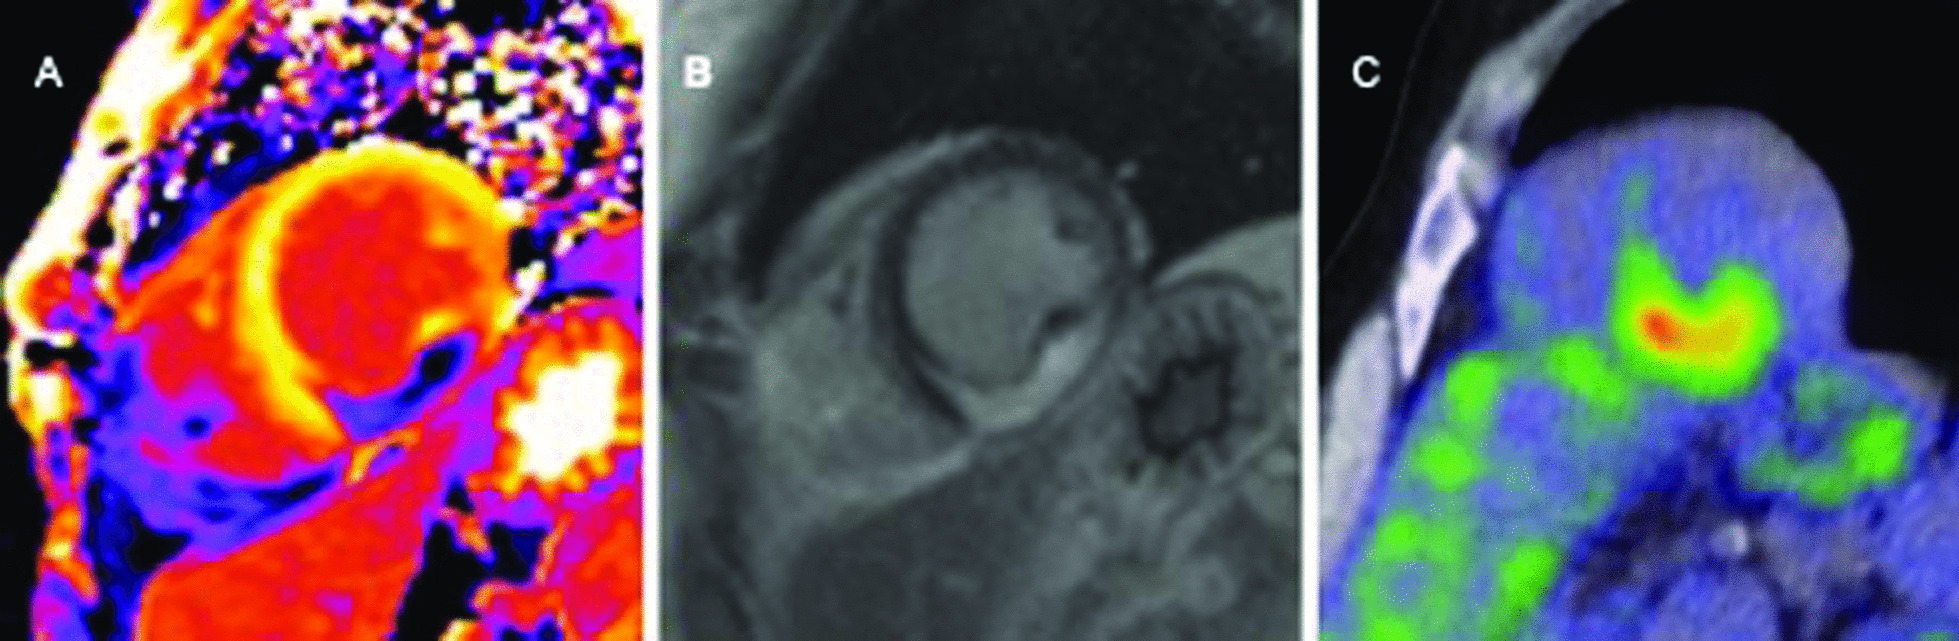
Example for determination of active cardiac sarcoidosis. Imaging findings in one patient with cardiac sarcoidosis (CS) in cardiovascular magnetic resonance (CMR) and 18-fluorodeoxyglucose positron-emission tomography-computed tomography (PET-CT). A Shows post contrast T1-map, B typical intense intramural Late gadolinium enhancement (LGE) and C pathological 18-fluorodeoxyglucose-uptake in PET-CT
